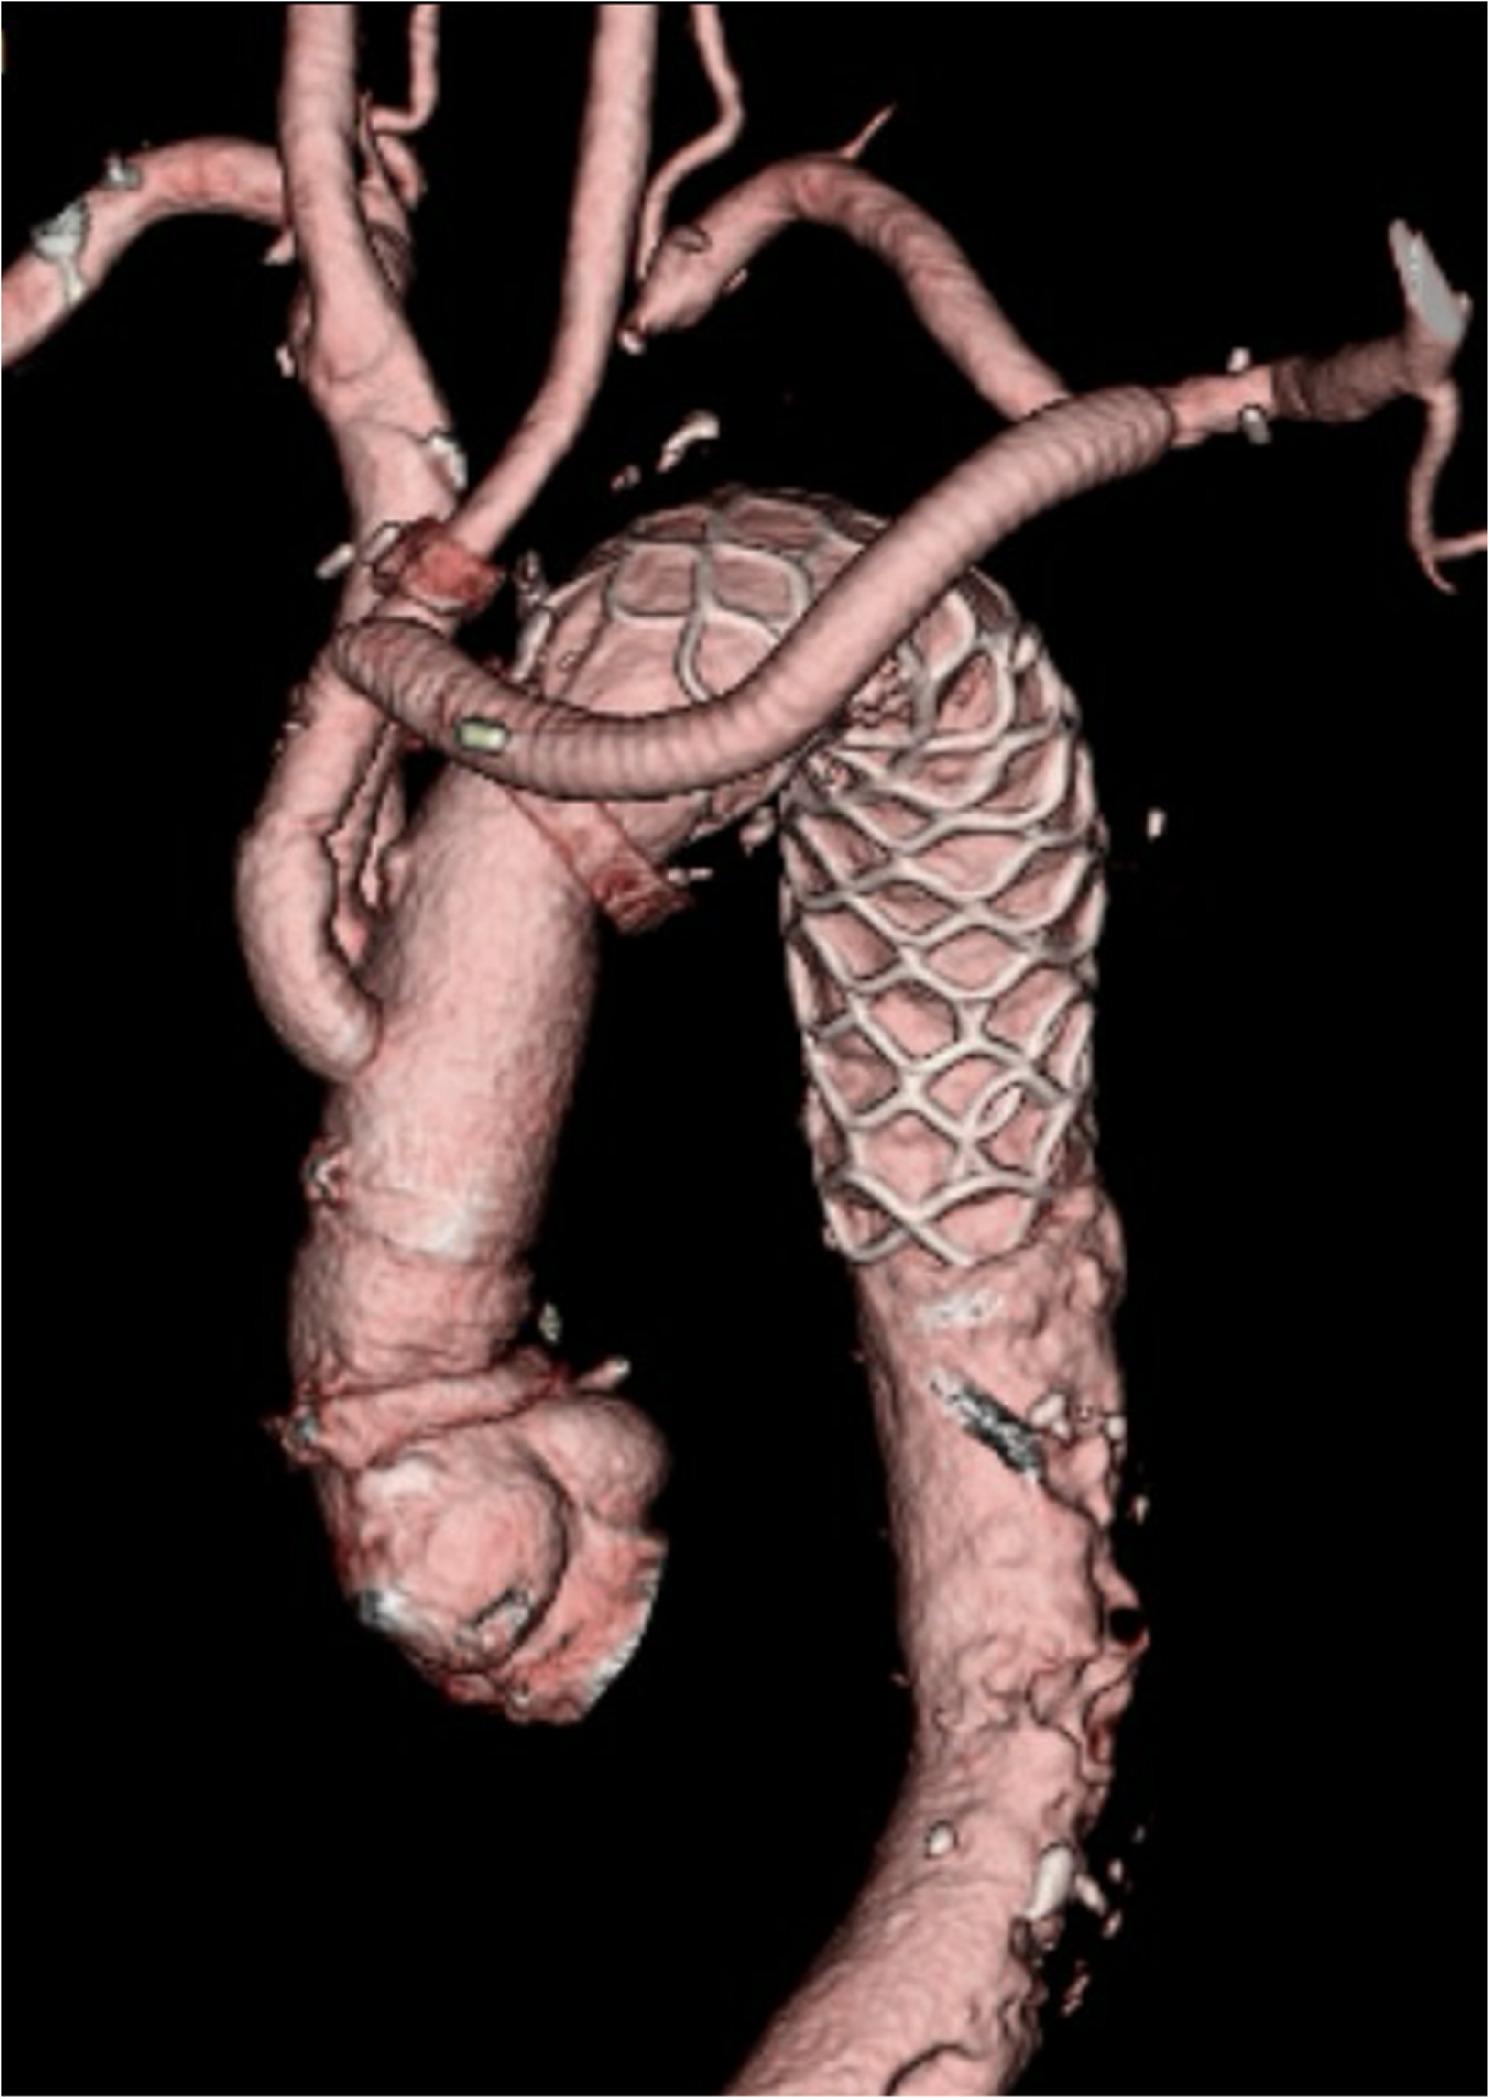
Postoperative contrast-enhanced computed tomography demonstrated complete exclusion of the aortic arch aneurysm without evidence of endoleak
